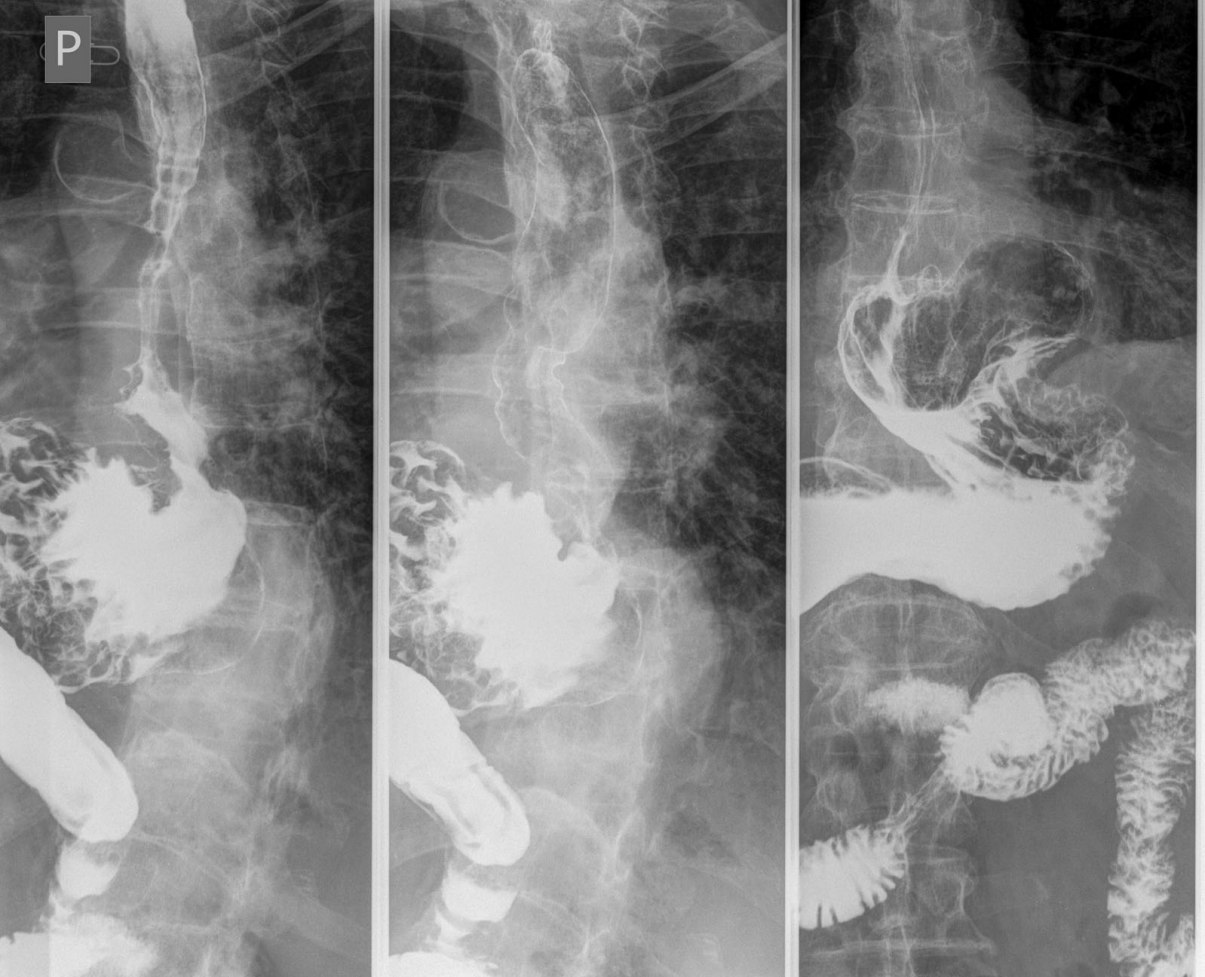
Features of sliding hiatus hernia in x-ray barium study
